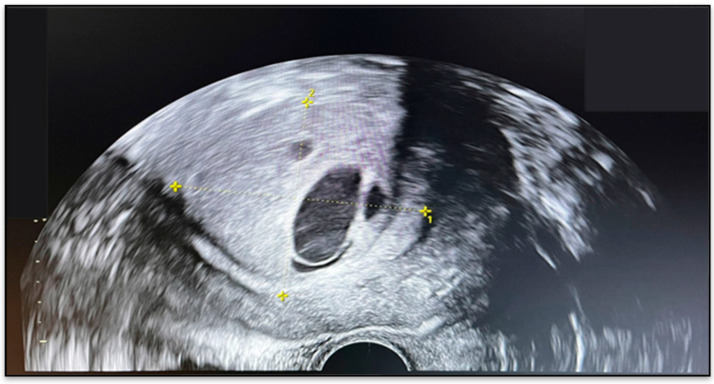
Transvaginal ultrasound showing an intracavitary uterine mass.
